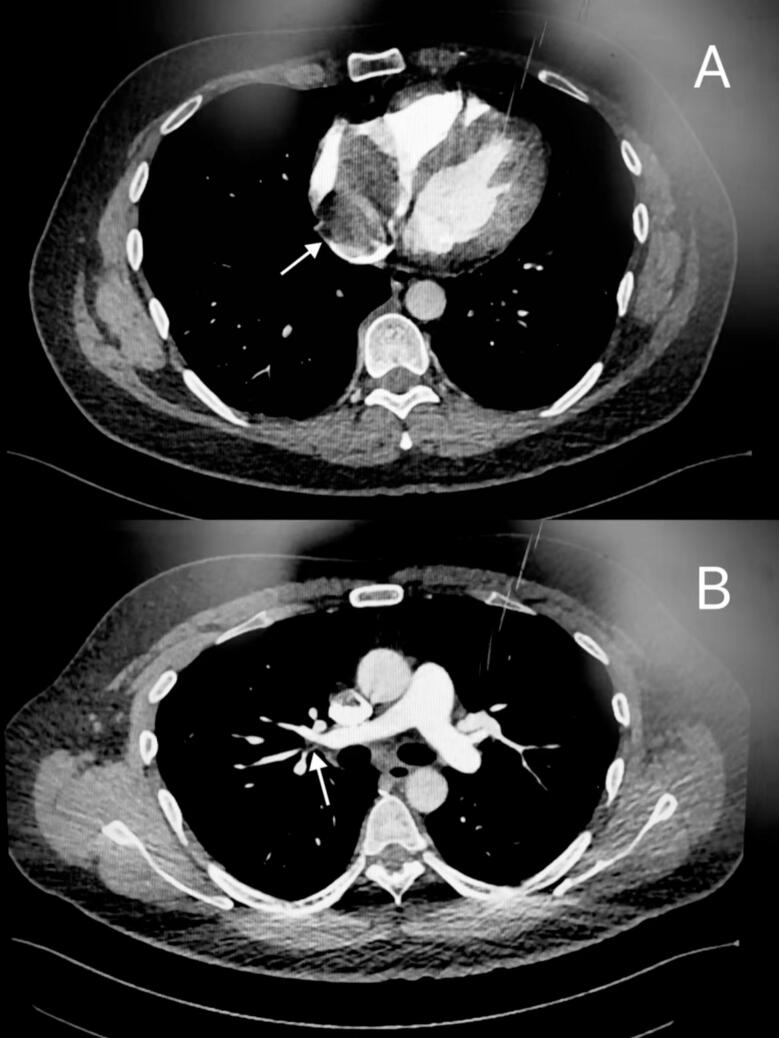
CT pulmonary angiography cross-section shows a massive irregular mass in the right atrium (A) and vascular embolism in the right lower lobe pulmonary artery branches (B).
